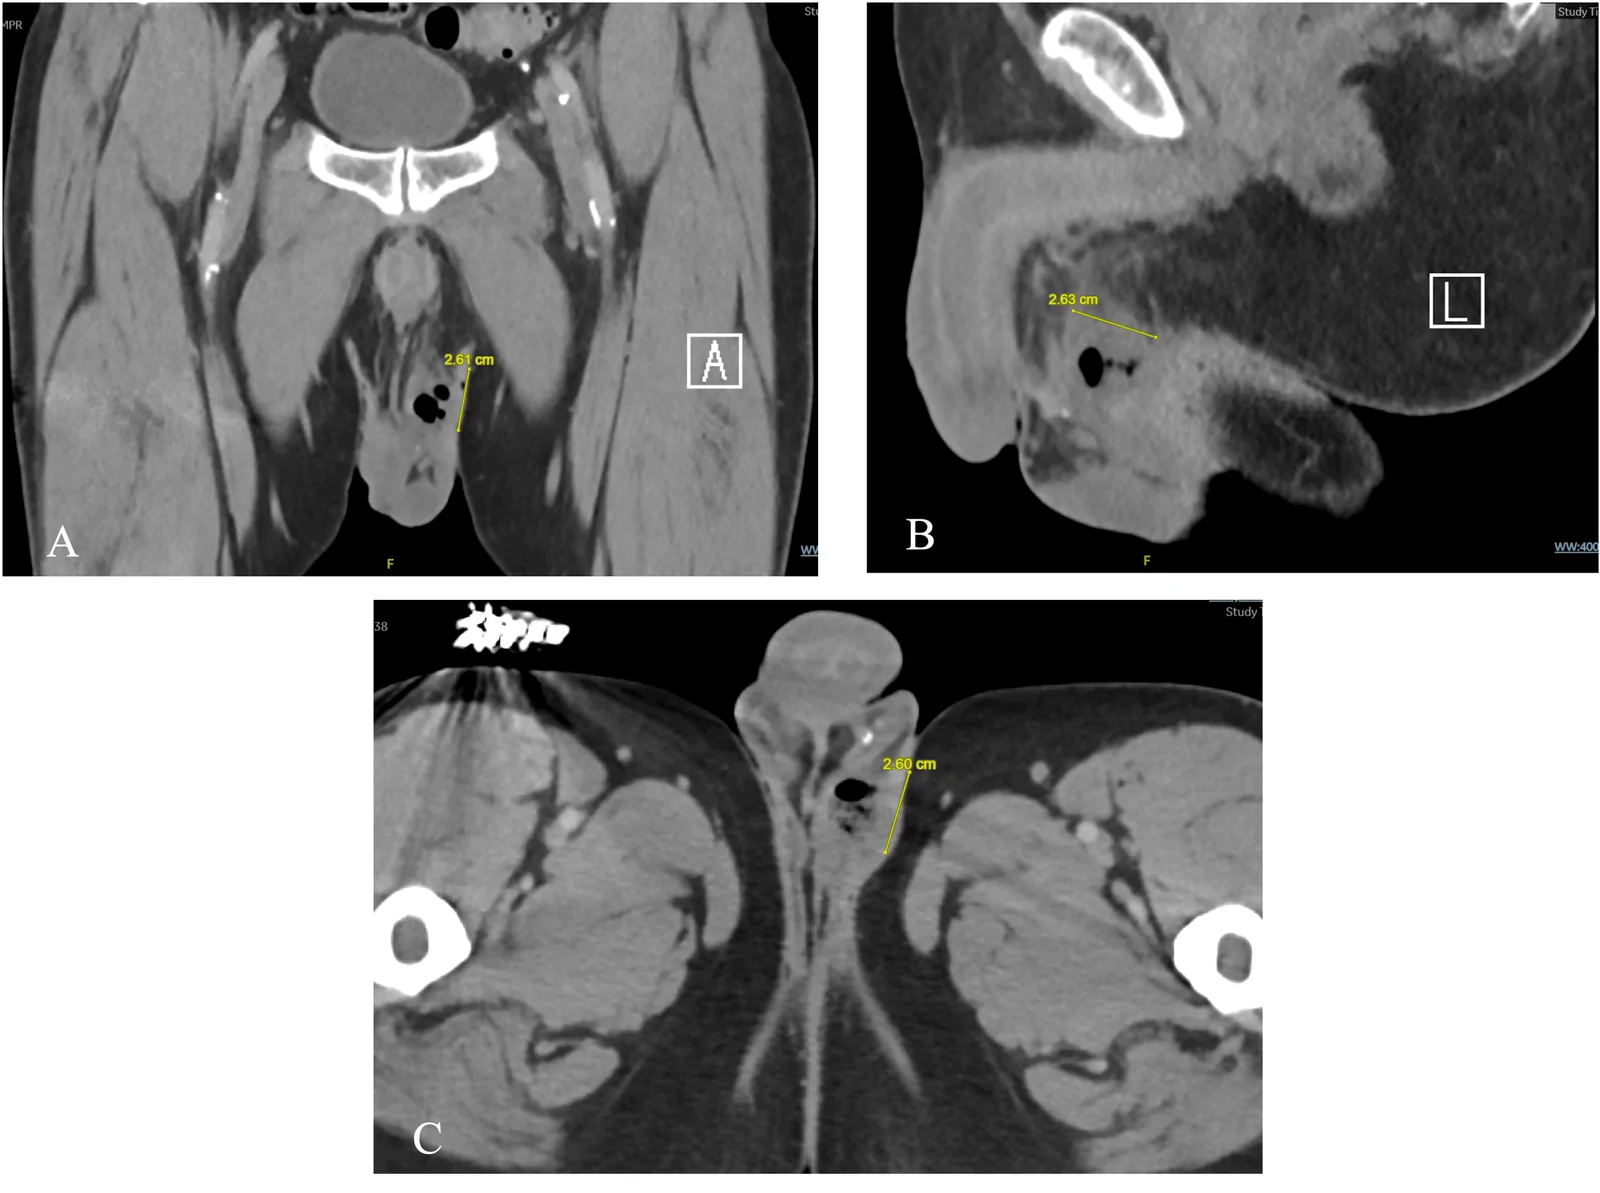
CT of the abdomen/pelvis with contrast showing a 2.6-cm gas-containing left hemiscrotal subdermal collection indicative of infection in coronal (A), sagittal (B), and transverse (C) views.
